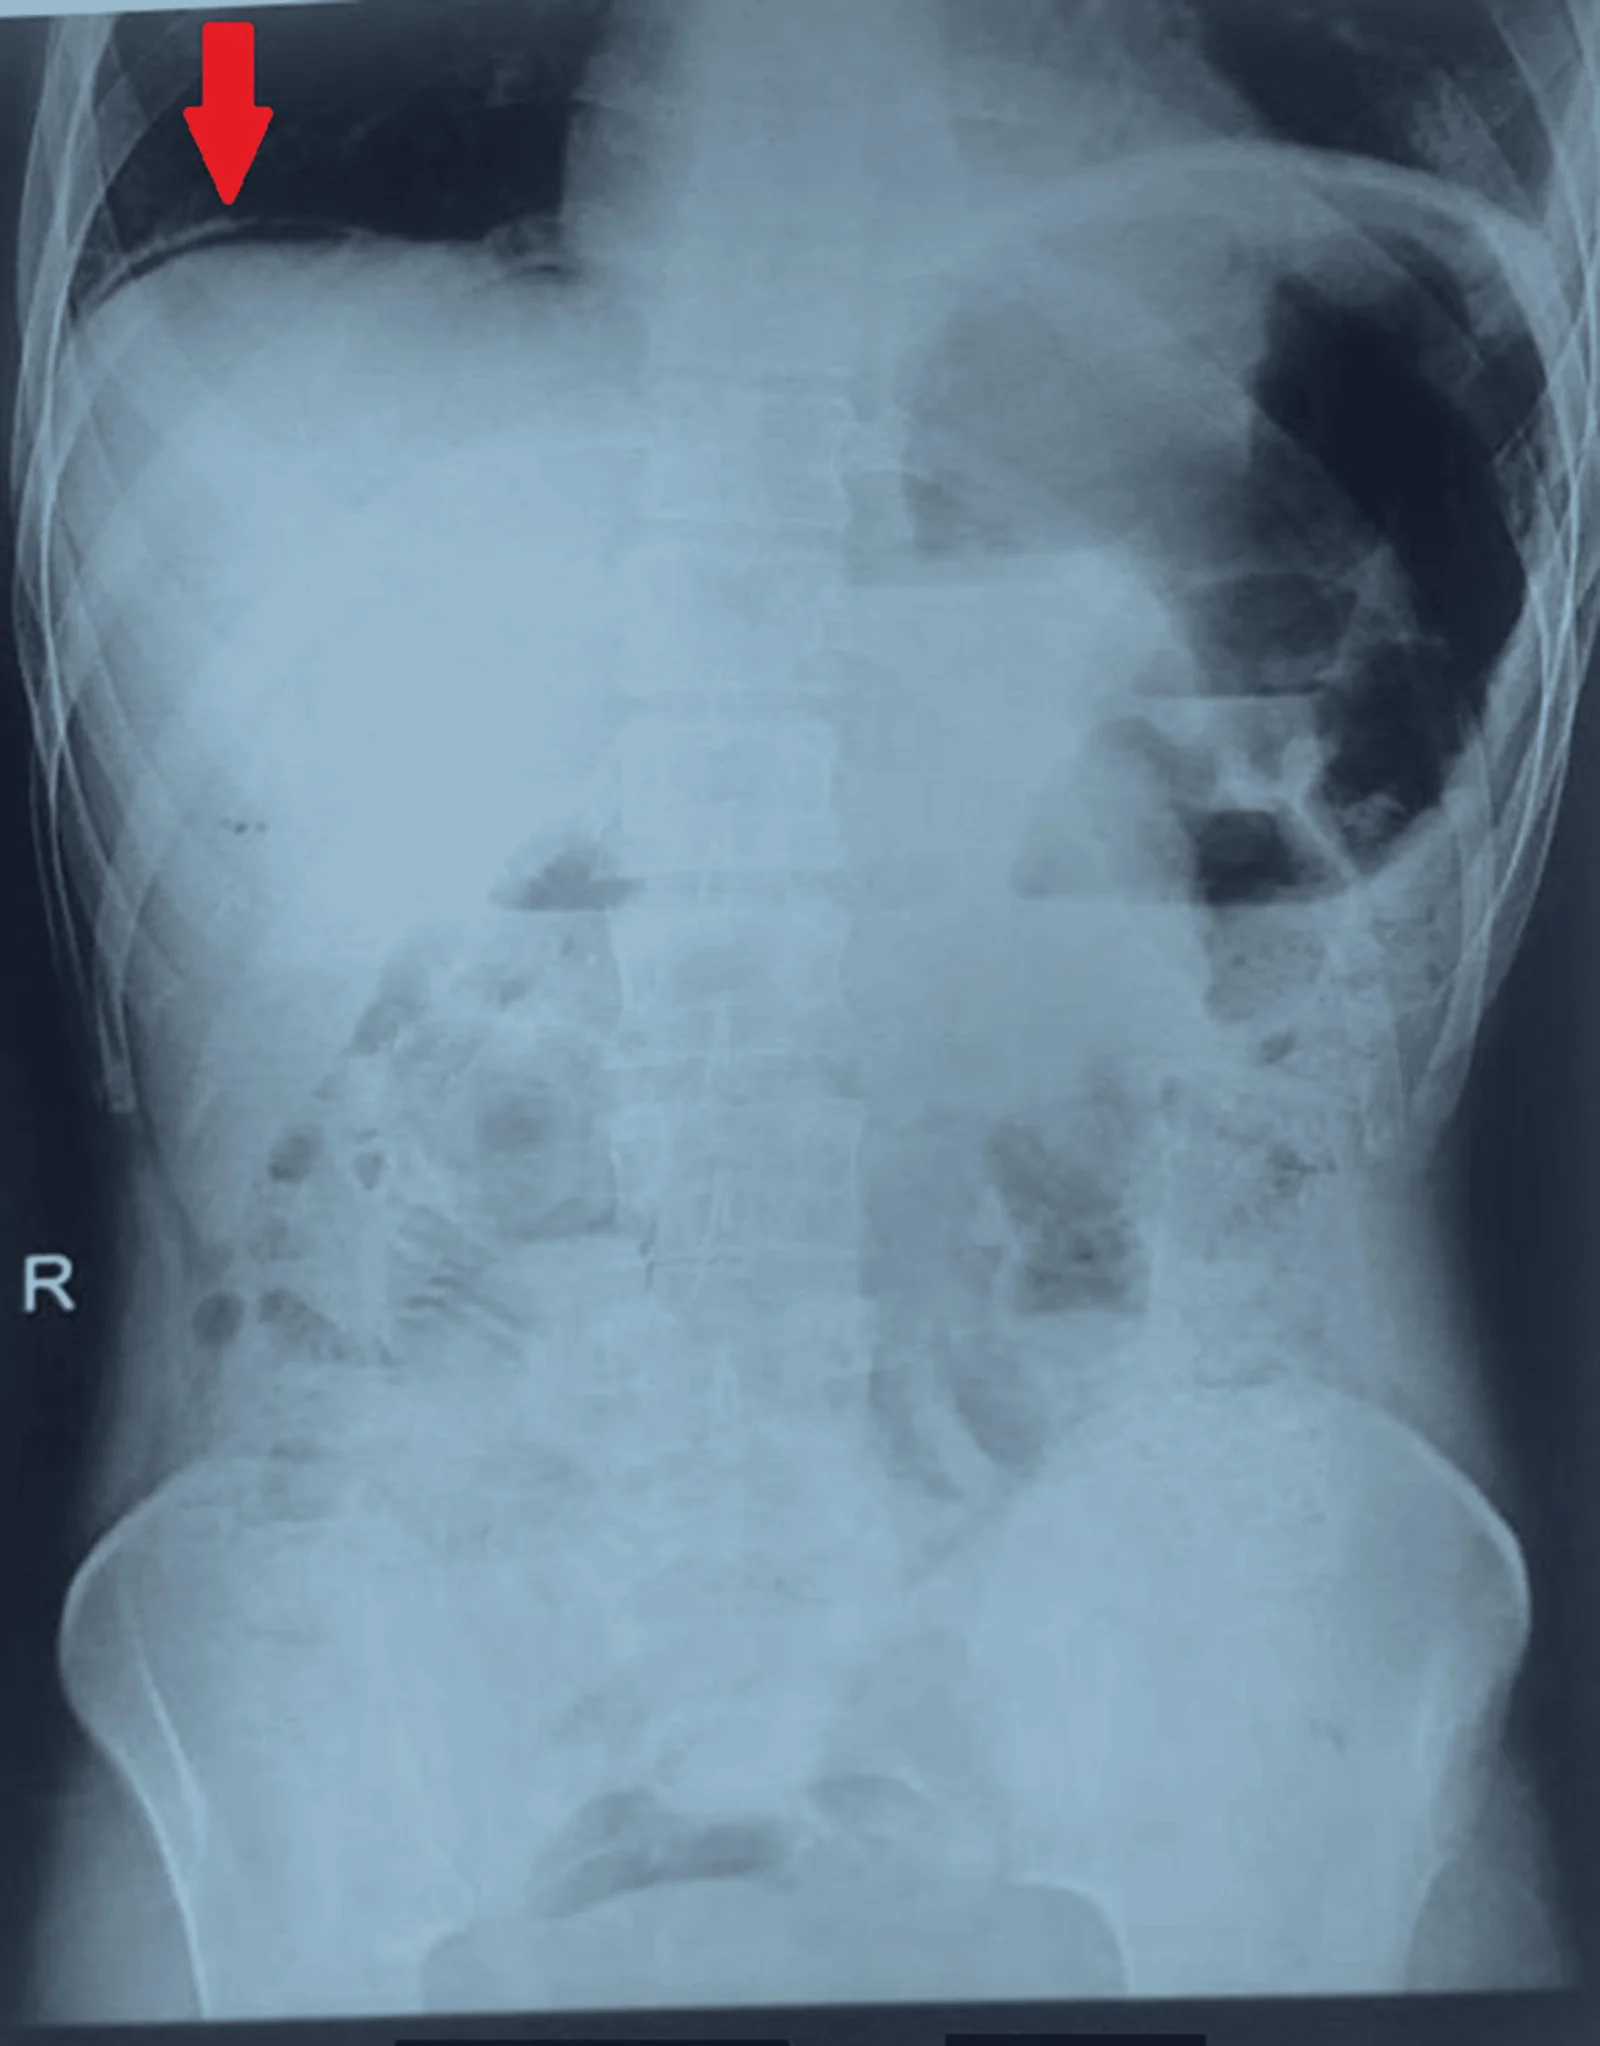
Erect abdominal X-ray showing gas under the diaphragm, suggestive of pneumoperitoneum (red arrow).
